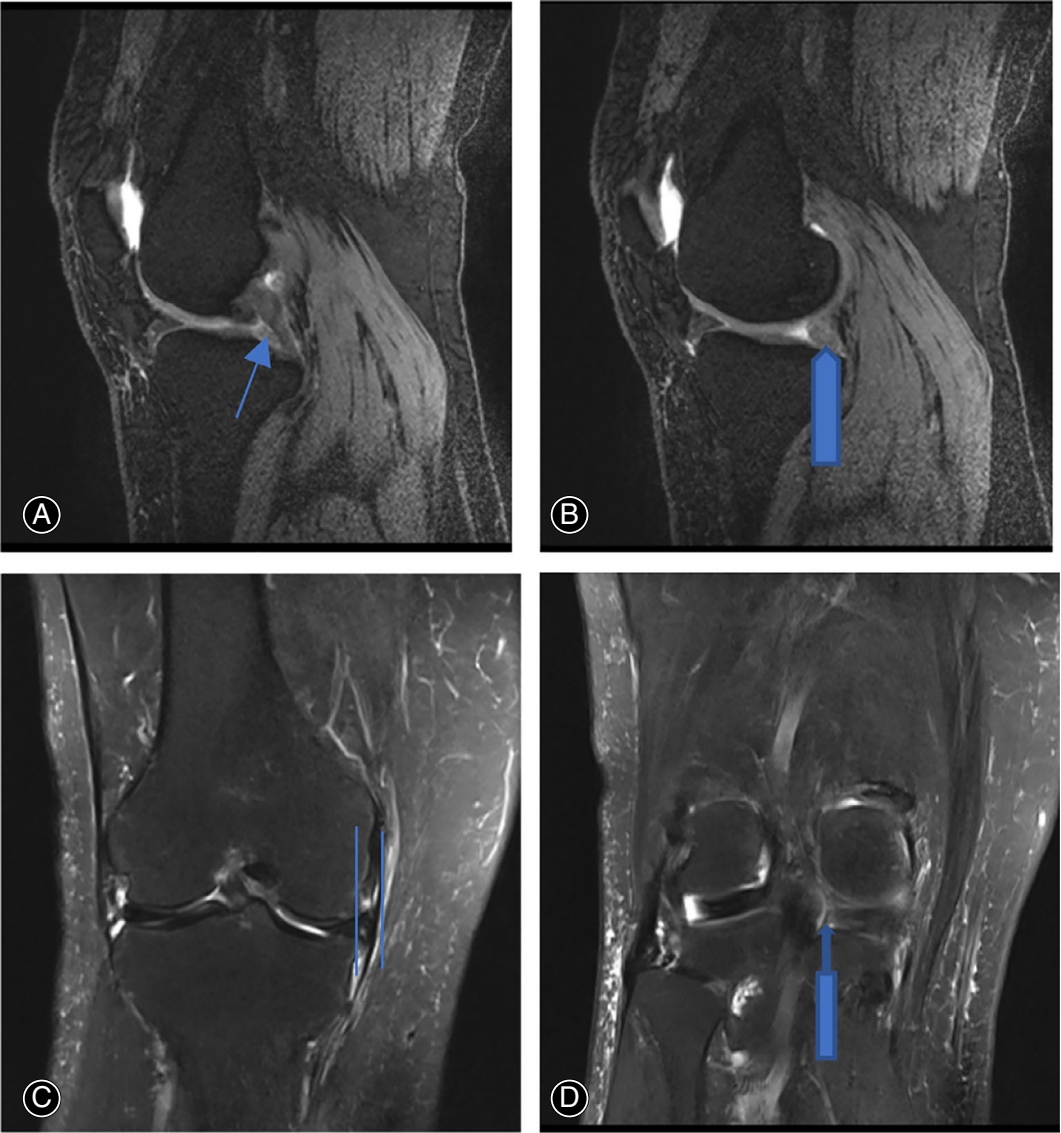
Preoperative MRI images of a 67‐year‐old woman patient with right knee MMPRT and normal limb alignment. (A) Ghost sign in T2 sagittal view indicated by long fine arrow (B) round shape (not as normal triangular shape) of medial meniscus posterior root in T2 sagittal view indicated by long coarse arrow (C) medial meniscus extrusion in T2 coronal view indicated by two lines (D) left sign in T2 coronal view indicated by long coarse upper arrow.
